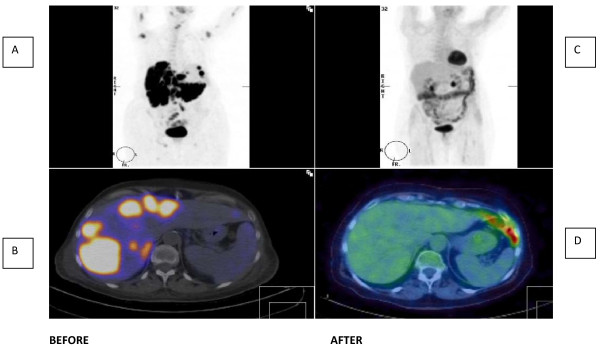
PET scan imaging before and after six cycles of R-CHOP. (A) PET scan before chemotherapy. Note multiple metabolically active areas in the liver, axillae, neck and base of skull. (B) PET-CT scan before chemotherapy demonstrating widespread metastases in liver. (C) PET scan after chemotherapy; complete resolution of metastases with only physiological appearances in gut. (D) PET-CT scan after chemotherapy showing complete resolution of liver metastases.
